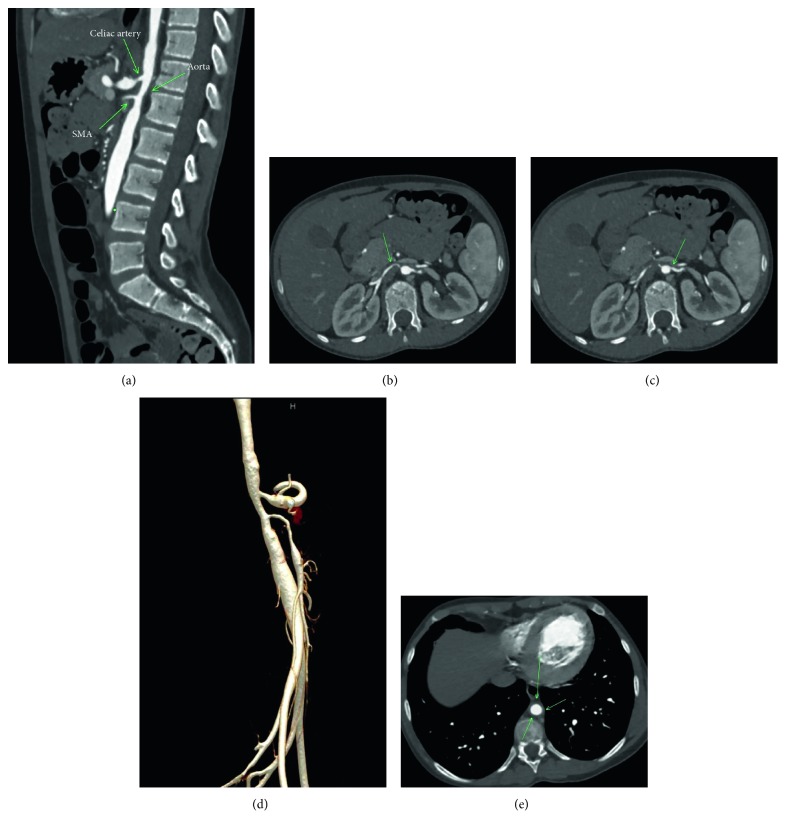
(a) CTA abdomen and pelvis showed narrowing of the mid-aorta, proximal renal arteries, celiac artery, and superior mesenteric artery; (b) CTA abdomen and pelvis showed narrowing of the mid-aorta and right proximal renal artery; (c) CTA abdomen and pelvis showed narrowing of the mid-aorta and left proximal renal artery; (d) CTA abdomen and pelvis showed narrowing of the mid-aorta, proximal renal arteries, celiac artery, and superior mesenteric artery; (e) CTA abdomen and pelvis showed narrowing of the mid-aorta.
